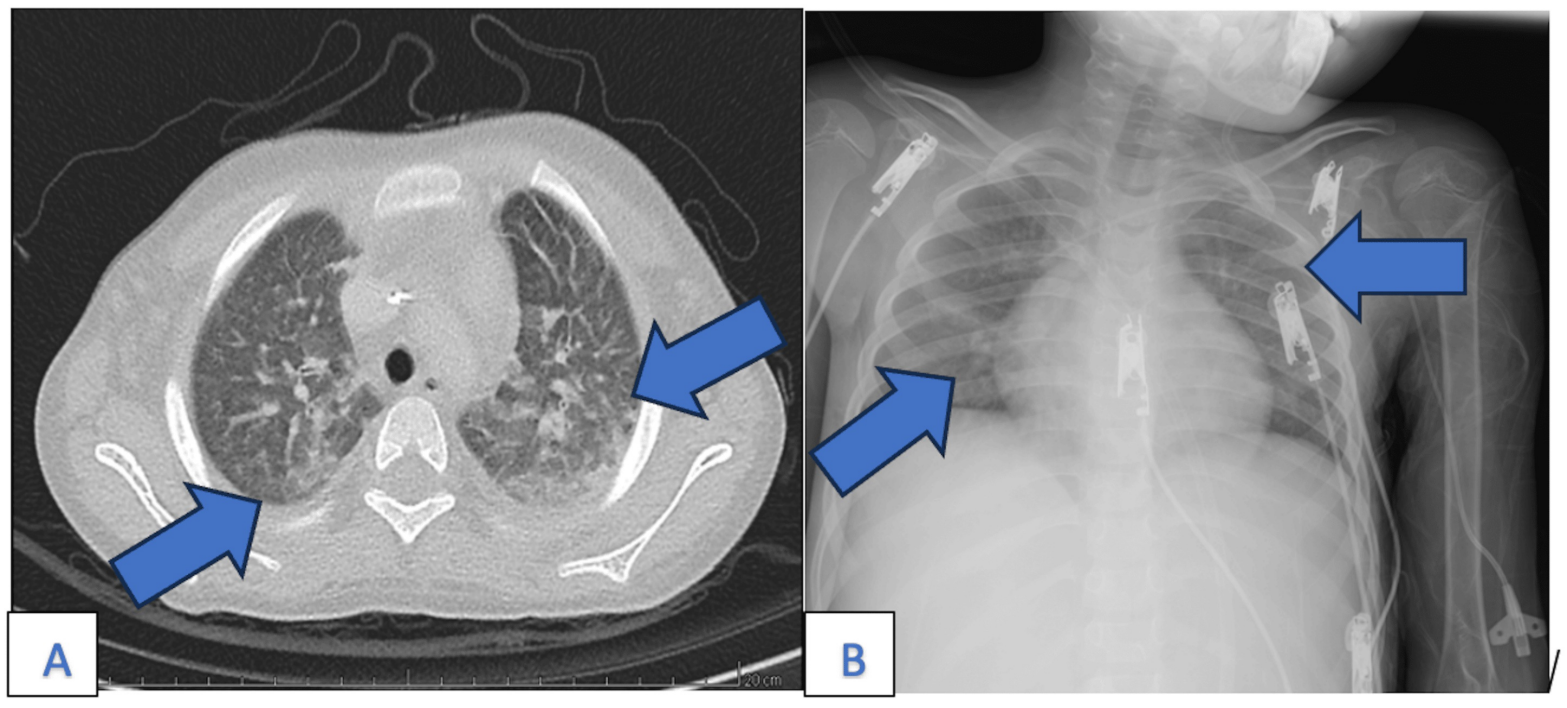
Chest CT and X-ray showing interstitial pulmonary involvement A: chest CT scan (axial view) showing bilateral diffuse ground-glass opacities (blue arrows), suggestive of interstitial pneumonia and raising concern for an opportunistic infection; B: frontal chest X-ray showing diffuse bilateral interstitial infiltrates with no focal consolidation (blue arrows). Portable imaging was limited but contributed to assessing disease monitoring.
